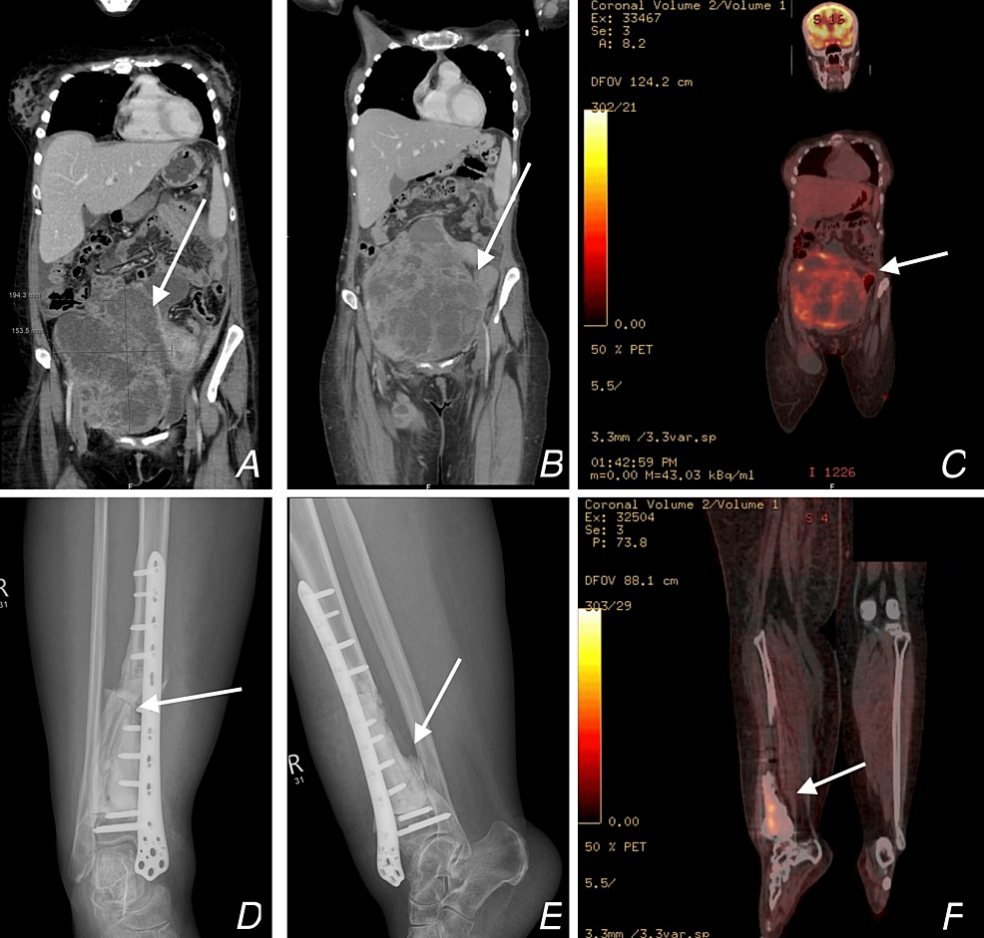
CT CAP images A: Coronal view of CT CAP measuring a pelvic mass of about 19.4 cm x 15.3 cm, pointed at by the arrow. B: Coronal view of CT CAP  showing the pelvic mass, pointed at by the arrow.  C: PET scan of the abdomen and pelvis, arrow showing the mass uptake of fluorodeoxyglucose. D: Antero-posterior (AP) view of the right distal tibia, the arrow of the distal tibial shaft displaced fracture of the cement. E: Lateral view of the right distal tibia, the arrow shows the distal tibial shaft displaced fracture of the cement. F: PET scan of the right tibia shows uptake of fluorodeoxyglucose. CT CAP: Computed tomography of chest, abdomen and pelvis, PET: Positron emission tomography.
